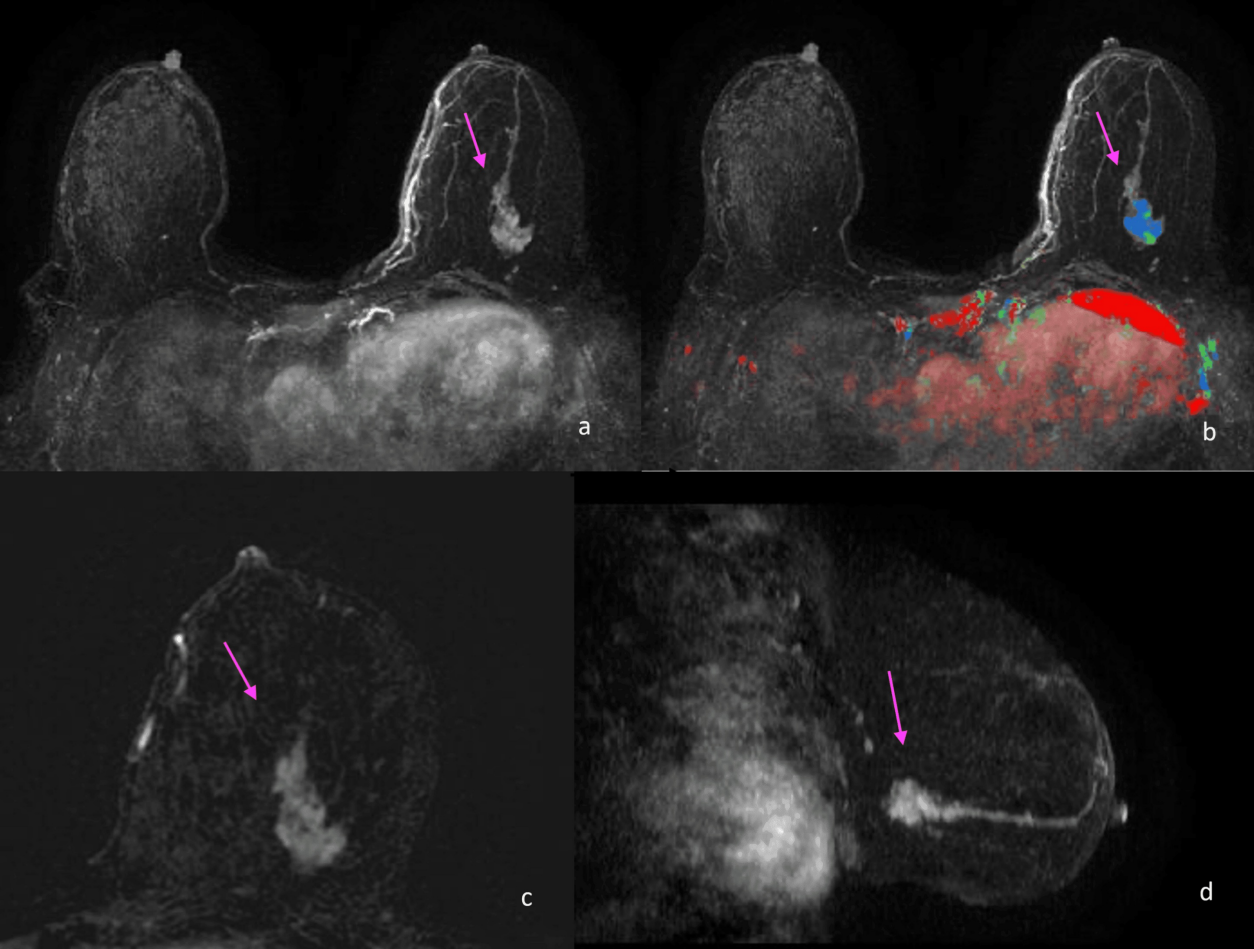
Contrast-enhanced MRI showing a 3.3 cm enhancing mass with irregular margins in the left breast. T1-weighted contrast-enhanced subtracted MRI axial (a-c) and sagittal (d) images obtained in November 2024 demonstrate a 3.3 cm enhancing mass with irregular margins in the inferior lateral left breast, extending from the middle to the posterior depth (arrows). Mixed kinetic enhancement was seen within the mass (b).
